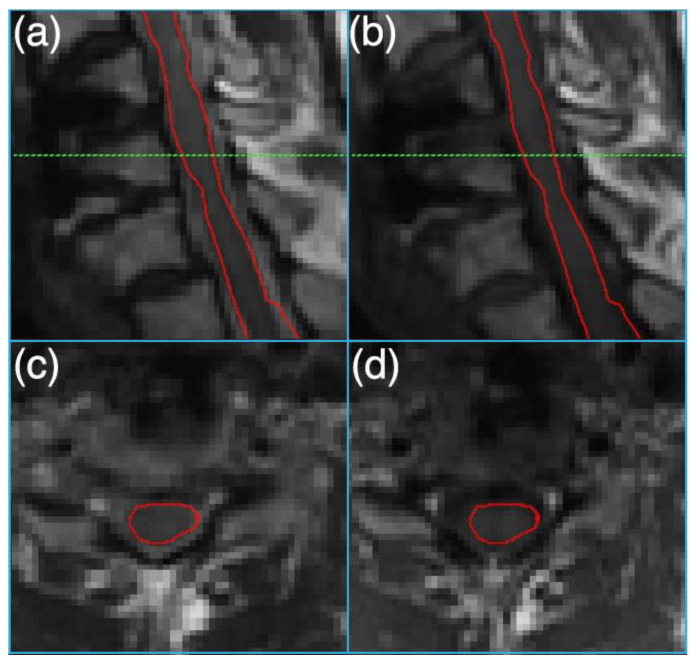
Sagittal (a) T_2_-weighted and (b) T_1_-weighted 3D-MSI MRI images of an instrumented damaged spinal cord. Axial sections, reformatted at the level of the dashed green line from (a,b), are shown in (c,d), respectively. The spinal cord is outlined in red in all images.
